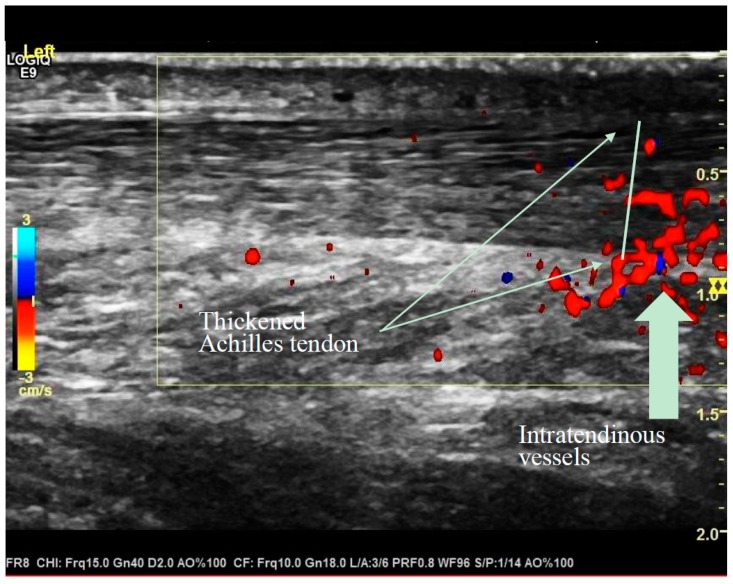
Thickened Achilles tendon and intratendinous vessels recorded at diagnostic ultrasound examination.
